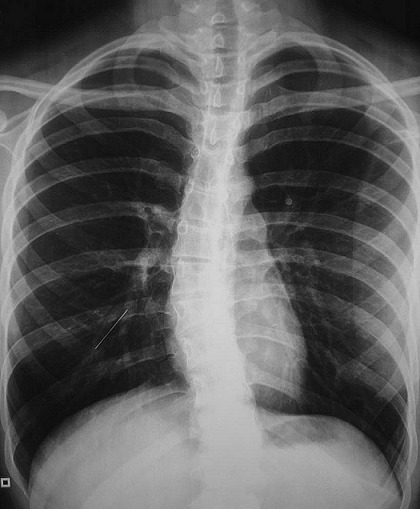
Radiographie thoracique de face montrant une opacité linéaire au niveau de la pyramide basale droite, correspondant à une épingle à foulard
